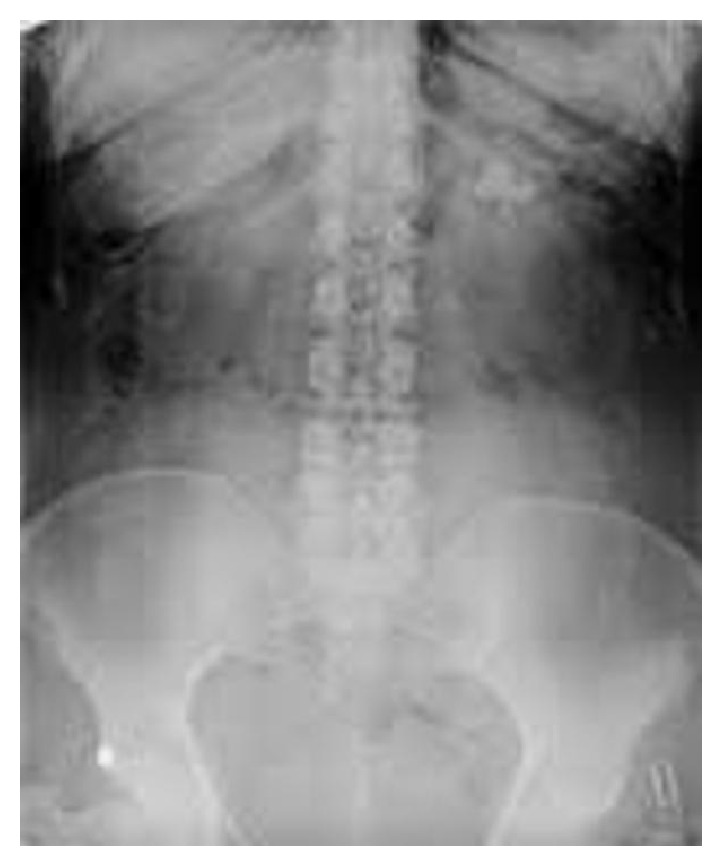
Preoperative plain X-ray of the kidney, ureter, and bladder region showing a partial staghorn left renal stone.
